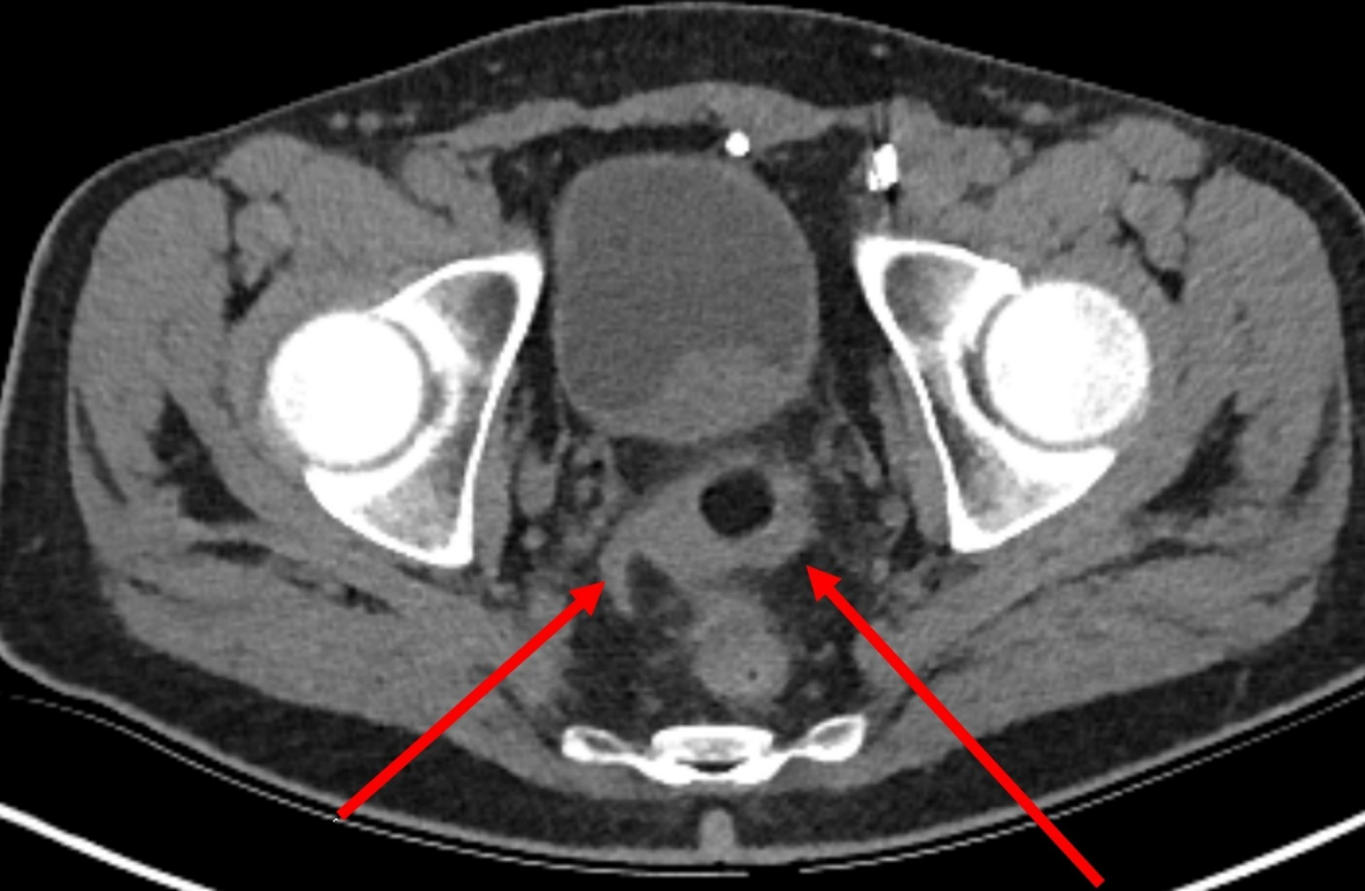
Computed tomography (CT) scan of the abdomen showing mild inflammation/fluid adjacent to distal sigmoid colon.
